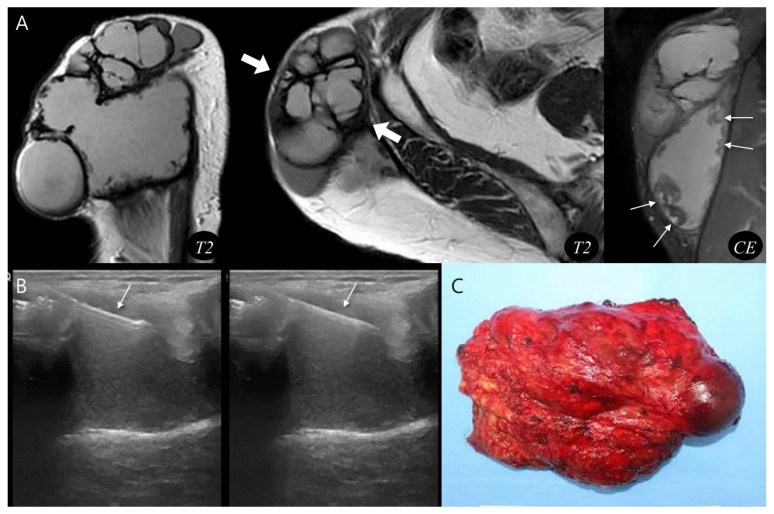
Diagnosis and treatment of chronic expanding hematoma. (A) Sagittal and axial T2-weighted MR images demonstrate a multilobular, subcutaneous mass (thick arrows) with internal septations. The lesion shows peripheral nodular enhancement (thin arrows, granulation tissue) on coronal T1-weighted contrast-enhanced MR image. (B) Ultrasound-guided biopsy was performed using a core needle (arrows), confirming the hematoma. (C) Gross photograph of the wide excision demonstrates chronic expanding hematoma.
